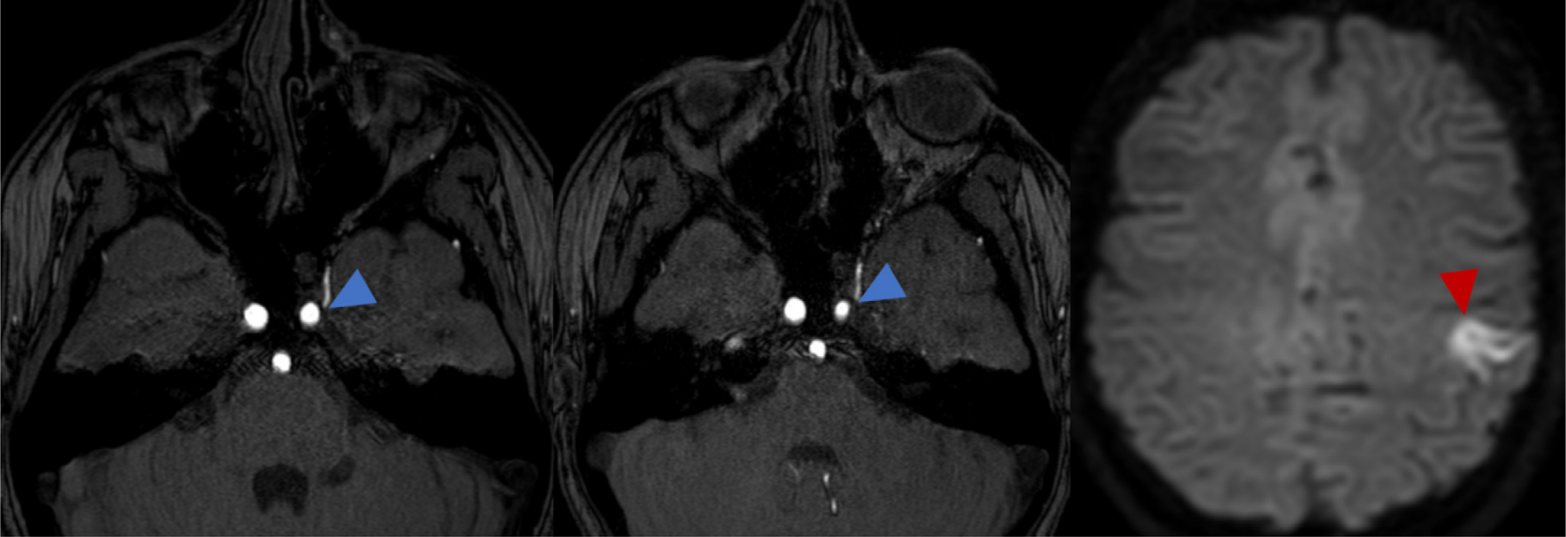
(A) Initial MRI brain before trametinib therapy with slightly small left ICA (blue arrow), and (B, C) MRI brain 9 months later after trametinib therapy with (B) progressive narrowing of left ICA (blue arrow) and (C) acute focal ischemia (red arrow).
